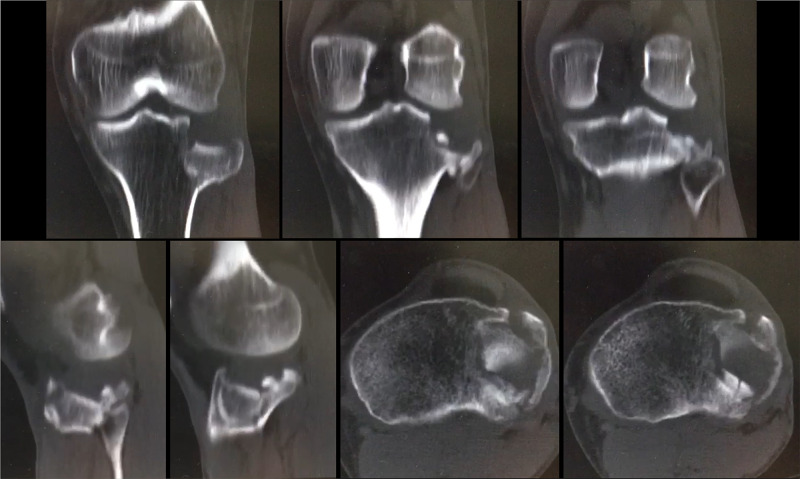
GM, 35-year-old, male patient, fall from a motorcycle Preoperative CT scan of the left knee – note the comminution at the depressed area, mainly on axial images CT: computed tomography
